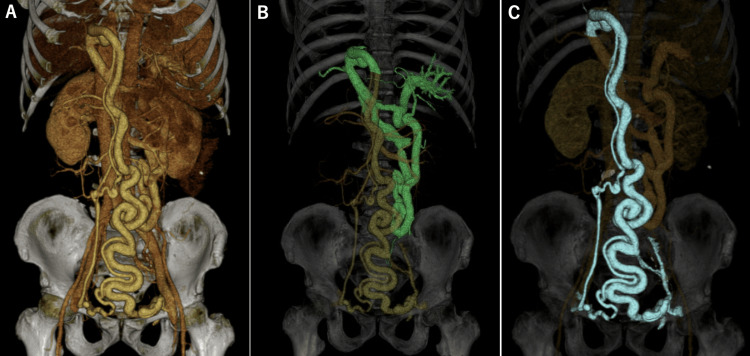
Three-dimensional volume-rendered CT images during the portal venous phase demonstrating two routes of the portosystemic shunt. (A) Overall view of both shunt pathways. (B) Mesorenal shunt (MRS), visualized in green. (C) Paraumbilical venous shunt extending from the left branch of the portal vein to the bilateral common femoral veins, visualized in blue.
